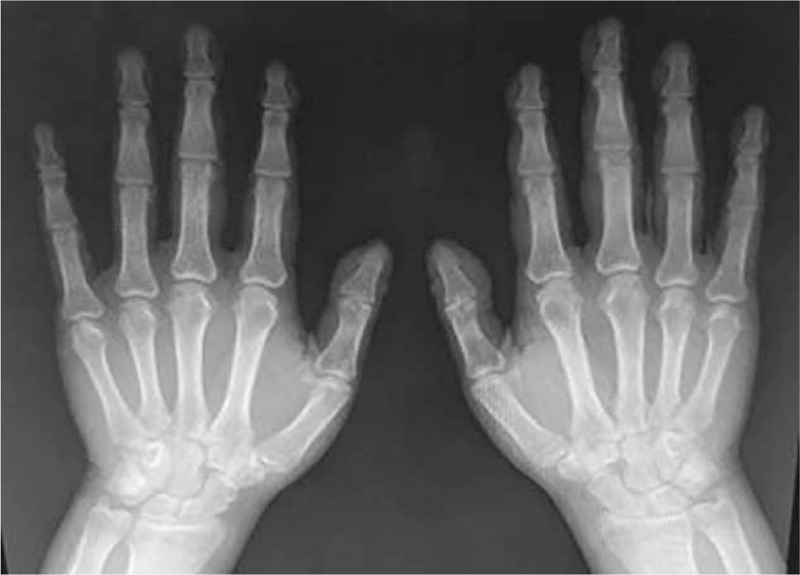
Radiographic examination of the upper limbs.
